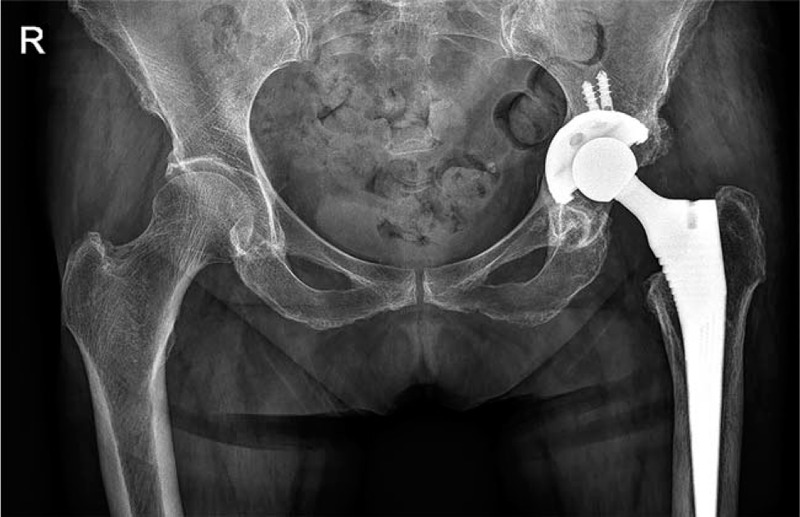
X-ray showed that changes had occurred after the total hip arthroplasty.
